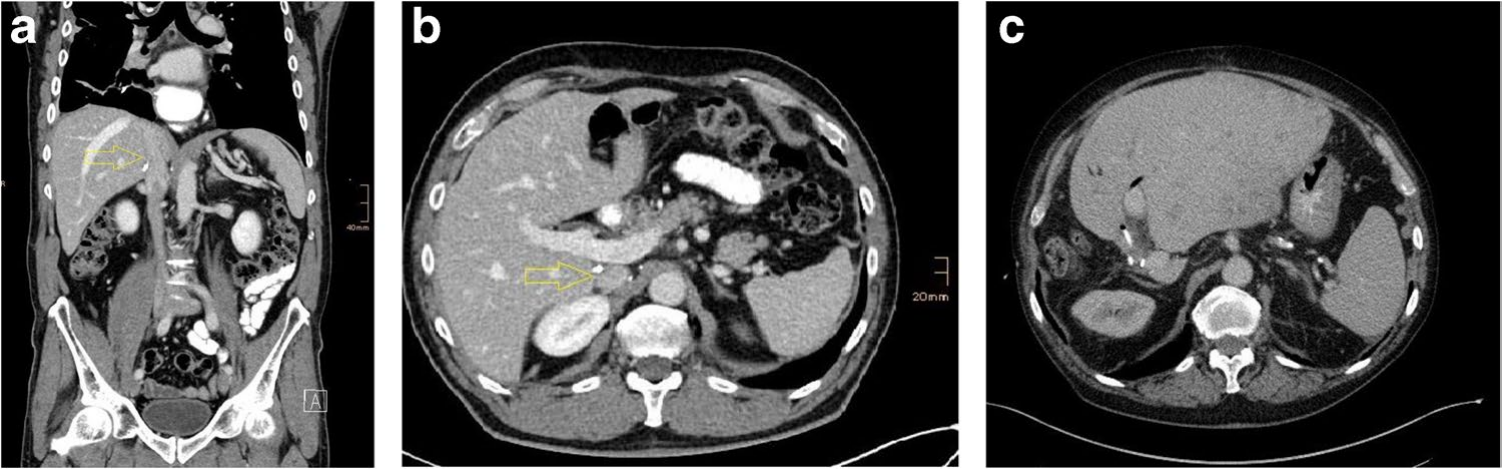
A and B CT scan of a patient 2 years after right hemihepatectomy with IVC resection and PPP reconstruction for colorectal metastasis without signs of obstruction, stenosis, or thrombosis; C CT scan showing good patency of VCI 4 years after reconstruction with PPP during right hemihepatectomy for colorectal metastasis
